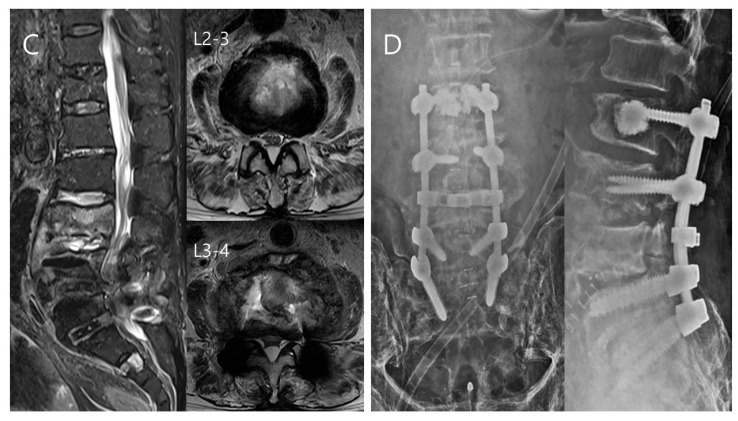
A 70-year-old female presented with worsening low back pain and radiating pain from the left buttock to the posterior thigh. She was diagnosed with L4–L5–S1 spinal stenosis and underwent partial laminectomy, posterior lumbar interbody fusion, and posterior instrumentation at L4–L5–S1. Four months after surgery, she presented to the hospital with sudden onset of severe back pain, and examination of the surgical site revealed swelling, fluctuance, and warmth. We suspected a late postoperative infection, which was confirmed by X-ray (A) and computed tomography (B). There was no implant loosening finding, such as osteolysis around screws on the radiologic evaluations; she underwent first incision and drainage surgery a week after the SSI diagnosis without a toothbrush, which resulted in temporary improvement. However, during the next 2 months, she was noted to have persistent signs of postoperative infection, and imaging studies showed spondylodiscitis, destruction of the upper vertebra endplate, and progression of the kyphotic deformity (C). She eventually underwent revision surgery with screw removal and additional instrumentation for extended fusion to L2; her postoperative X-rays are shown in (D). The patient provided informed consent to publish all of her clinical images.
